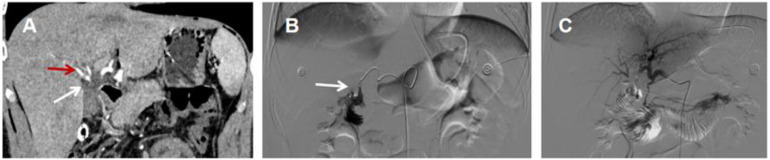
(A) Enhanced abdominal CT showing the right hepatic artery (red arrow) adjacent to the choledochojejunostomy anastomosis (white arrow); (B) Celiac arteriography confirming a rupture in the right hepatic artery with contrast leakage, directly communicating with the choledochojejunostomy anastomosis (white arrow) and (C) Repeat angiography after embolization of the right hepatic artery showing no contrast leakage, with loss of right hepatic blood flow and well-preserved left hepatic artery and left liver perfusion.
